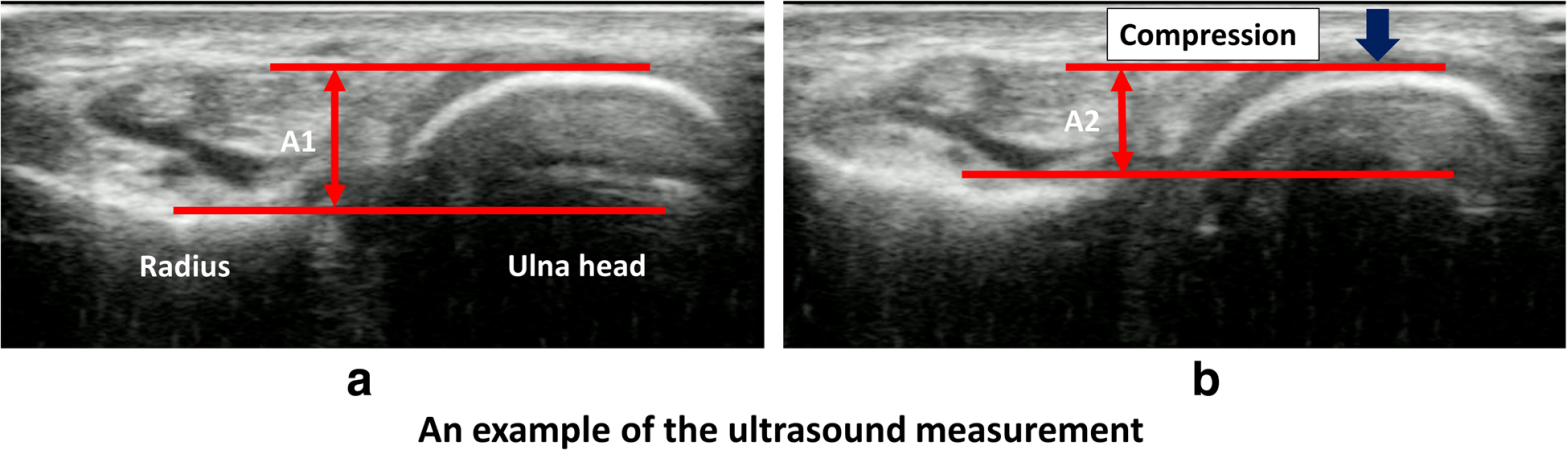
An example of an ultrasound measurement. a Initial position. A1: distance between the dorsal surfaces of the ulnar and the radius at the initial position. b Final position after compression. A2: distance between the dorsal surfaces of the ulnar and the radius at the final position after the compression. Arrow shows pressure to the ulna. A1–A2 were defined as the changes of radioulnar distance
